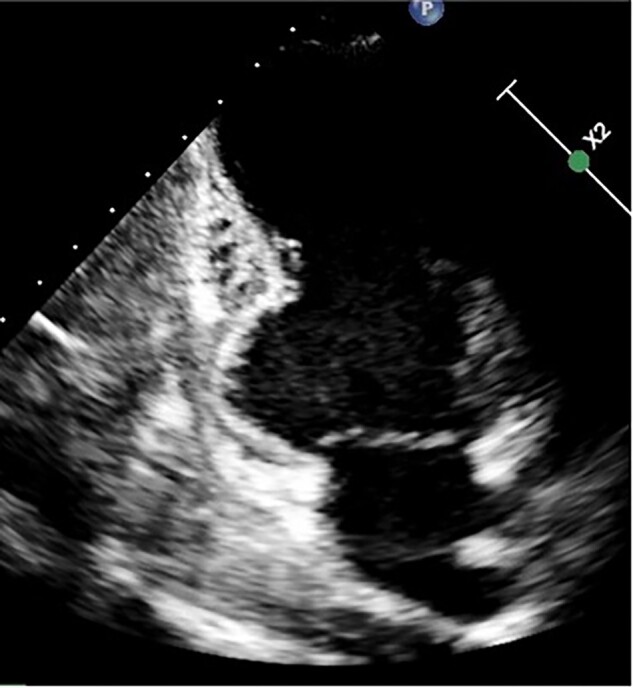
Patient 1, transthoracic echocardiogram: apical two-chamber view showing diverticulum of the inferior basal wall of the left ventricle (length 3.4 cm, depth 2.4 cm).
